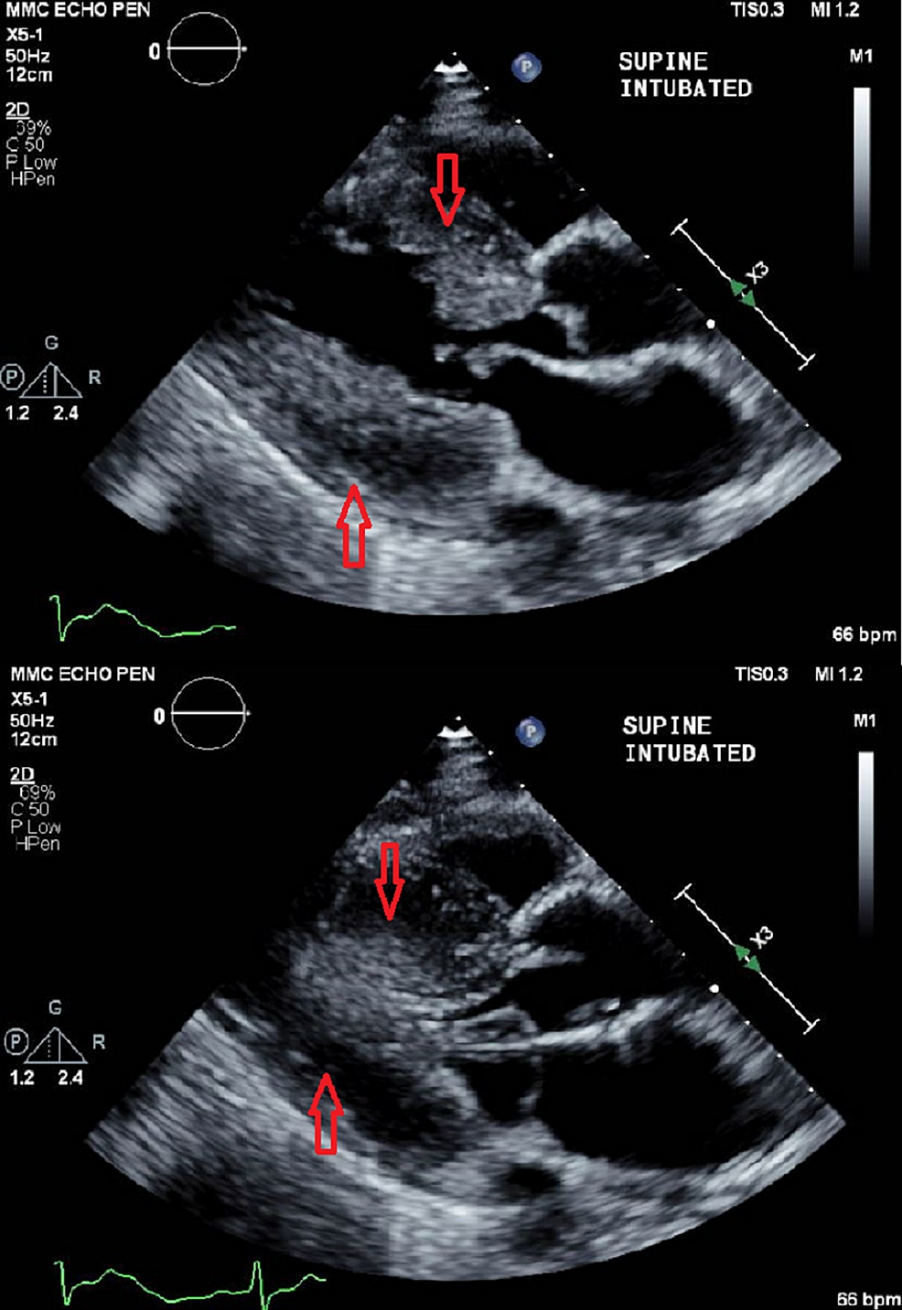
Transthoracic Echocardiogram Still images of a transthoracic echocardiogram obtained postoperatively to highlight the severity and extent of left ventricular hypertrophy and left ventricular cavity size during diastole and systole. Red arrows point to thickened anterior and inferior left ventricular walls.
